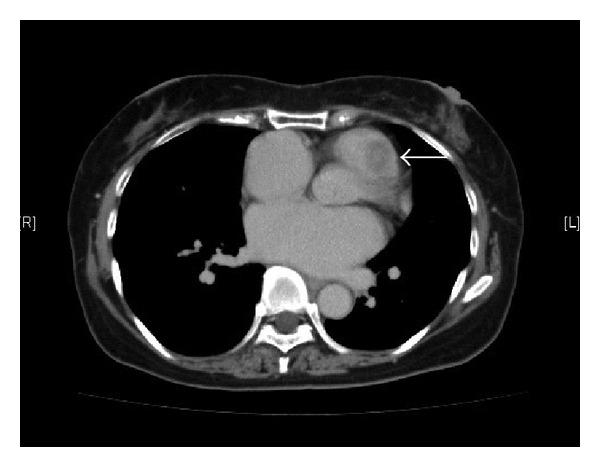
Chest computed tomography displays the tumor (white arrow) in the right ventricular outflow tract.
